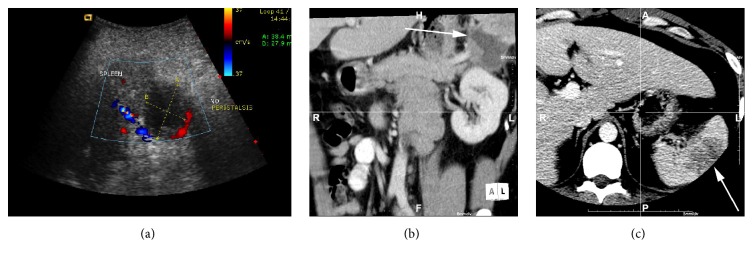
(a) Ultrasound scan demonstrating a pancreatic pseudocyst adjacent to the splenic hilum. (b) Coronal oblique reconstruction of contrast enhanced computed tomography. The arrow indicates the pancreatic tail pseudocyst, located at the splenic hilum. (c) Contrast enhanced computed tomography showing a heterogenous hypodense area in the spleen adjacent to the pancreatic pseudocyst. The white arrow refers to the spleen with hypodense area.
